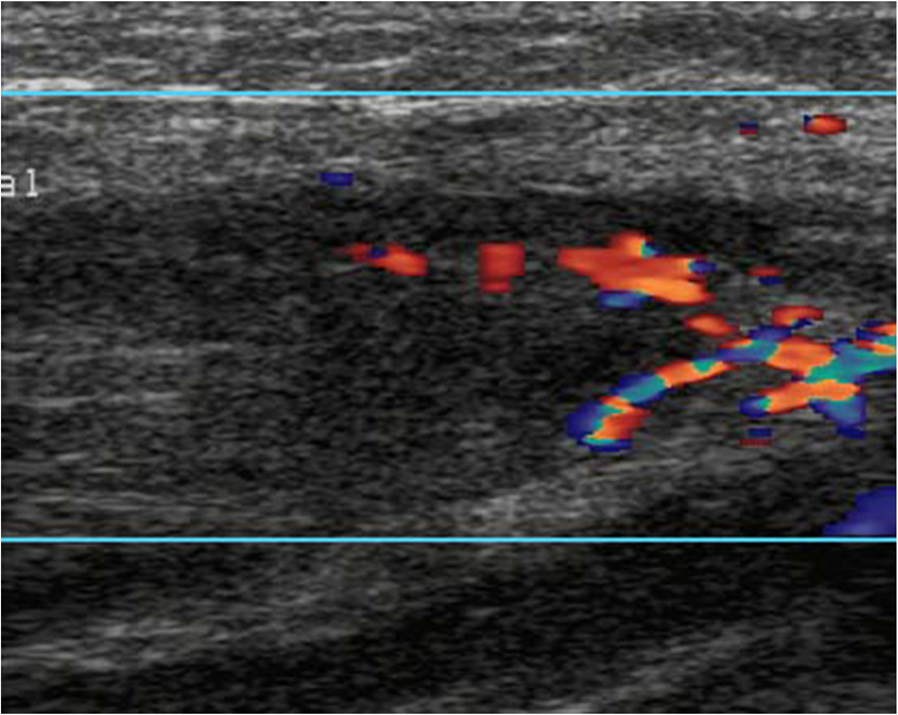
Ultrasonography of the right biceps brachii showed a hypo-echoic lesion within the muscle in which blood supply could be detected by color Doppler flow imaging.
